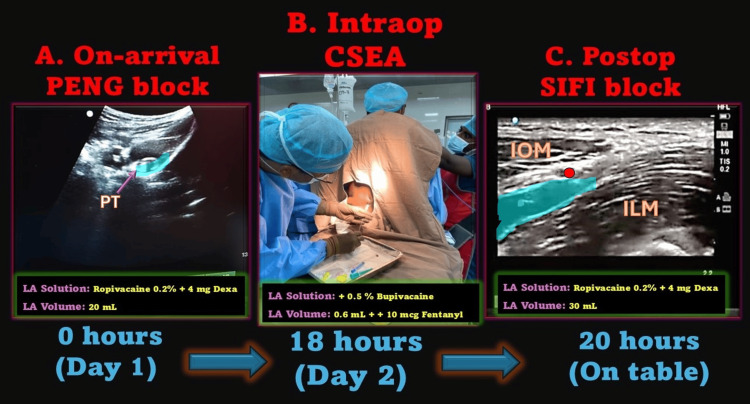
Perioperative regional anesthesia strategy in a 111-year-old supercentenarian. (A) On arrival, an ultrasound-guided pericapsular nerve group (PENG) block was performed using 20 mL of 0.2% ropivacaine with 4 mg dexamethasone, providing effective preoperative analgesia. The blue area shows local anesthetic spread under the psoas tendon (PT). (B) Intraoperatively, combined spinal-epidural anesthesia (CSEA) was administered with 0.6 mL of 0.5% hyperbaric bupivacaine and 10 µg fentanyl, achieving adequate surgical anesthesia while maintaining hemodynamic stability. (C) At the conclusion of surgery, a postoperative supra-inguinal fascia iliaca (SIFI) block was performed using 30 mL of 0.2% ropivacaine with 4 mg dexamethasone to ensure prolonged analgesia and facilitate early mobilization. The blue area denotes local anesthetic spread between the internal oblique (IOM) and iliacus muscle (ILM), under the fascia iliaca, located below the deep circumflex iliac artery (shown as a red circle). LA, local anesthetic; Dexa, dexamethasone; Intraop, intraoperative; Postop, postoperative Source: this figure was created by the first author, KS.
